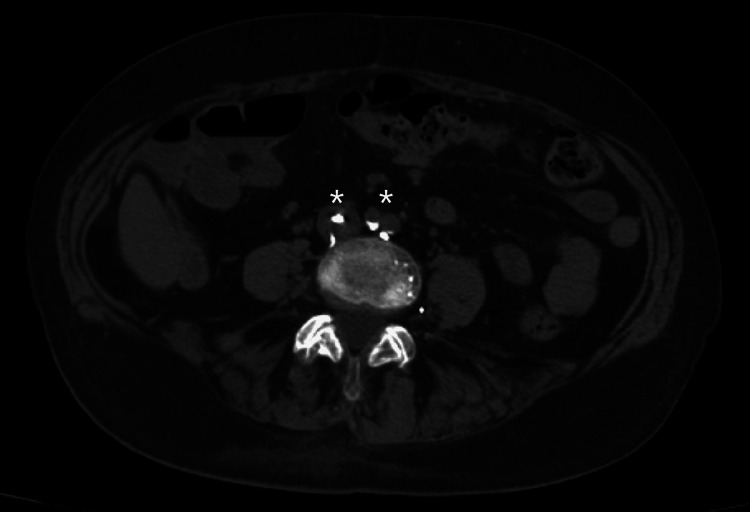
CT scan of the abdomen (axial cut) showing hyperdense emboli (asterisks) with the same density as the injected material in the repaired vertebra CT: computed tomography
